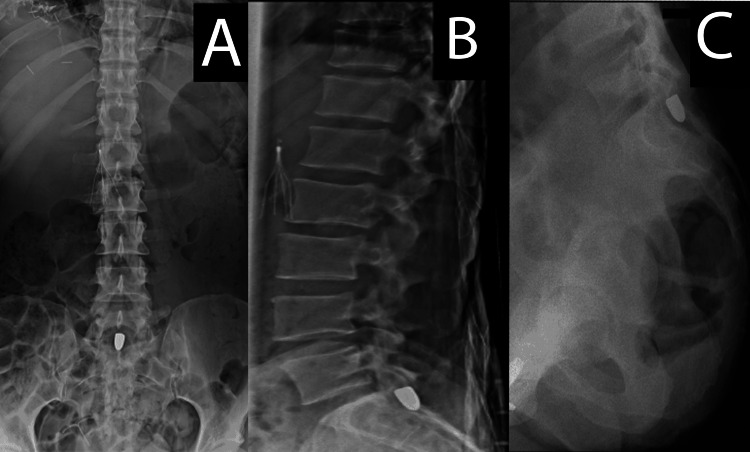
Spinal X-ray (A) Anteroposterior lumbar X-ray, (B) lateral lumbar X-ray, and (C) lateral sacral X-ray. The figure demonstrates the bullet position posterior to the S1 vertebral body.
